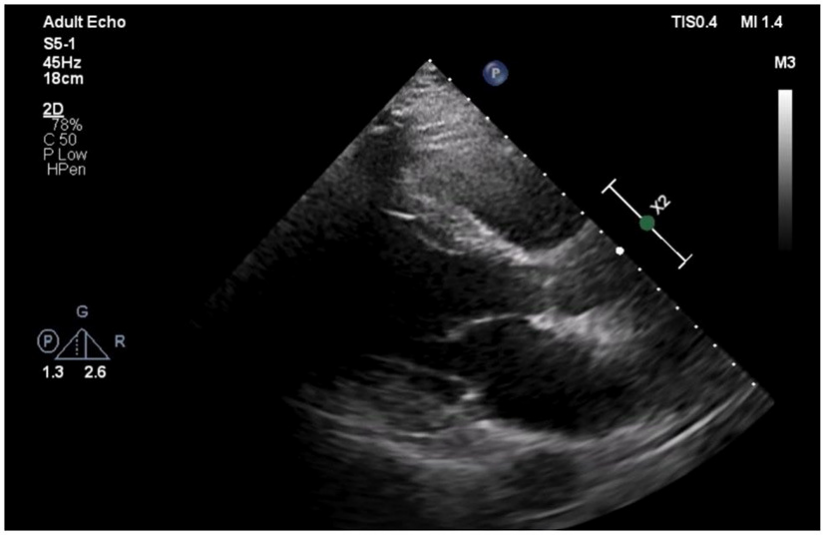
Echocardiogram of Left parasternal axis view shows no pericardial effusion.
